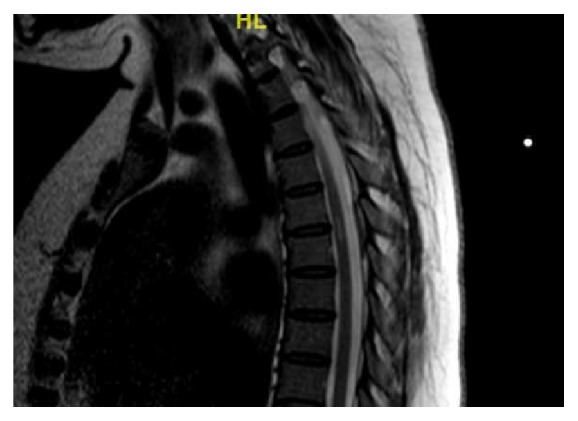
MRI T2 sagittal view of epidural blood collection which has decreased in size to 2-3 mm.
